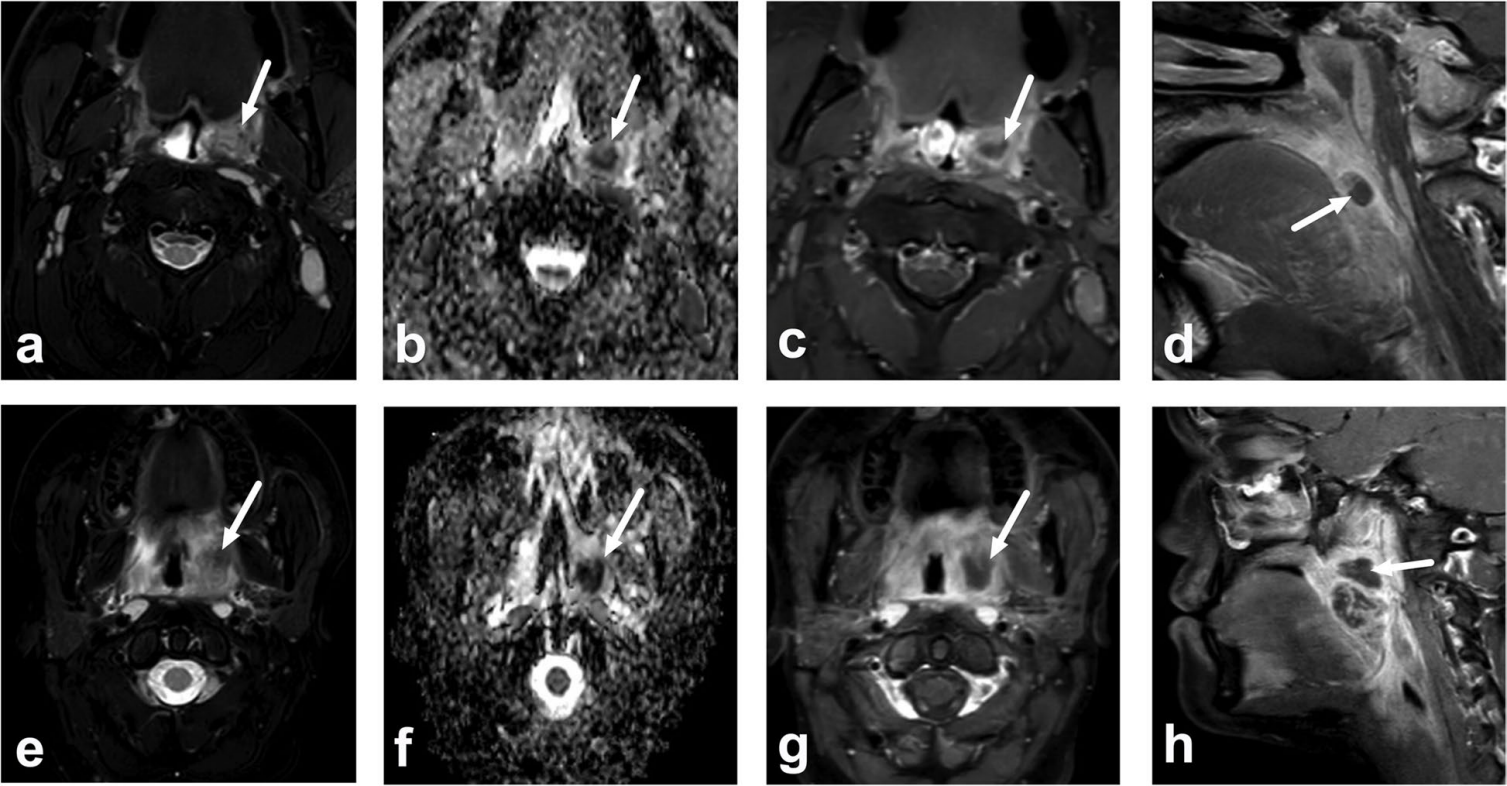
Examples of two difficult peritonsillar abscess (PTA) cases for the short protocol (a–d and e–h). Images are axial fat-suppressed T2-weighted images (a and e), apparent diffusion coefficient (ADC) maps (b and f), axial fat-suppressed postcontrast T1-weighted images (c and g), and sagittal postcontrast T1-weighted images (d and h). Both cases demonstrate areas of intermediate to low T2-signal (a and e) and low ADC (b and f) (arrows) in the region of the left palatine tonsil. Using the short protocol, one of three radiologists failed to identify the PTA in the first (top row), and all three radiologists missed the PTA in the second patient (bottom row), although the non-enhancing abscess (arrows in c, d, g, and h) was clearly identified using the full protocol by all readers. In both cases, abscesses is not well seen on T2-weighted imaging because of intermediate to low T2-signal, and low ADC can be mistaken for normal tonsillar tissue in patients with small palatine tonsils
